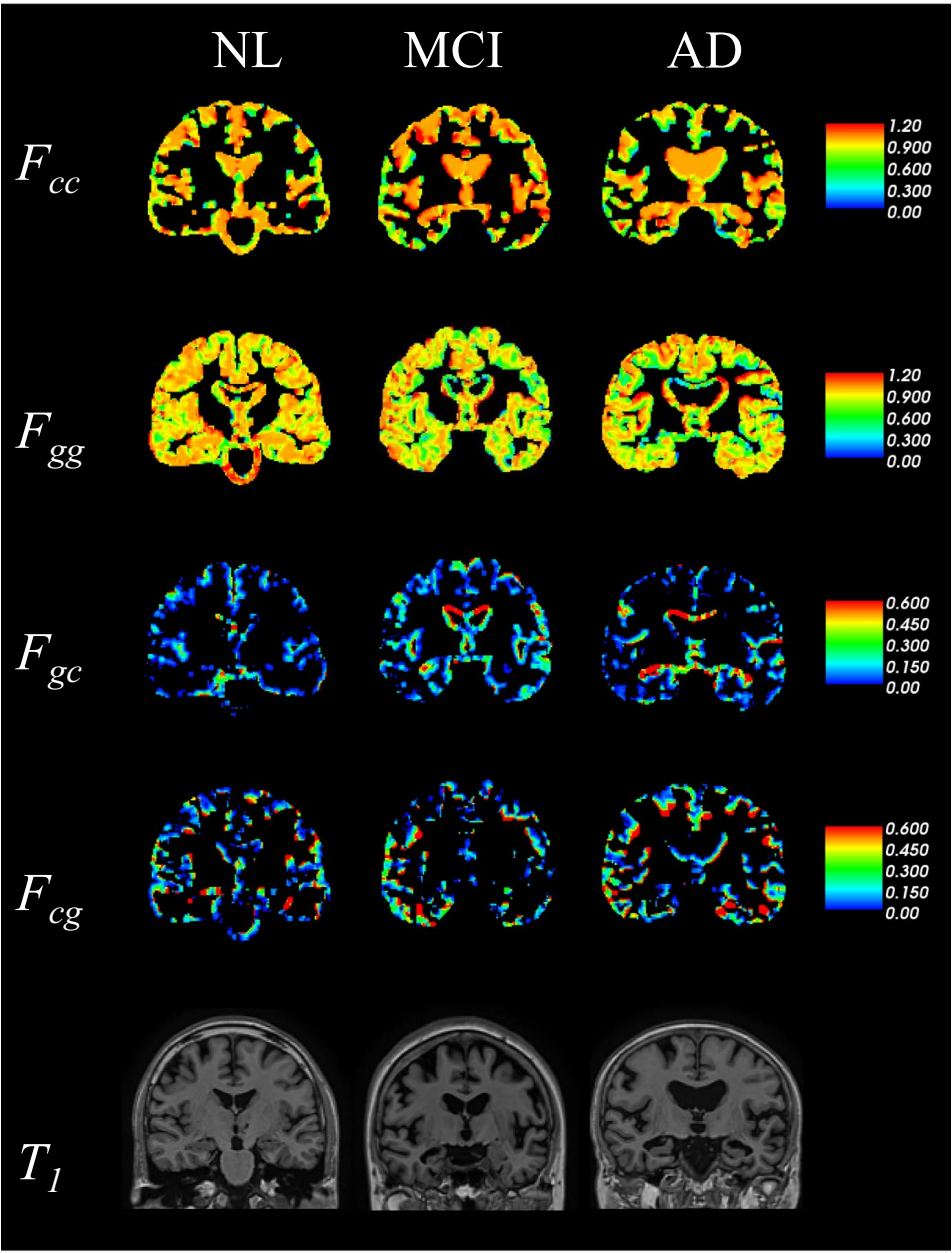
Representative images in NL (left column), MCI (middle column), and AD (right column) of each CPC element related to changes in GM and CSF (*F_cc_*, *F_gg_*, *F_gc_,* and *F_cg_*), and original T1‐weighted magnetic resonance image of SST. Color maps of image show CPC values. AD, Alzheimer's disease; CPC, coefficients of probability change; CSF, cerebrospinal fluid; GM, gray matter; MCI, mild cognitive impairment; NL, normal cognition
